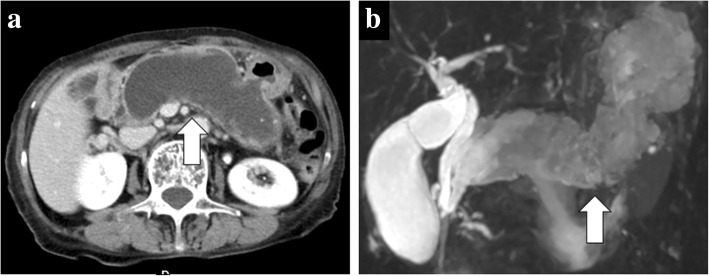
Dilatation of the main pancreatic duct in the entire pancreas. a Contrast-enhanced computed tomography revealed a markedly dilated main pancreatic duct (55 mm) and thinning pancreatic tissue (white arrow). b Magnetic resonance cholangiography revealed main pancreatic duct dilatation (white arrow)
